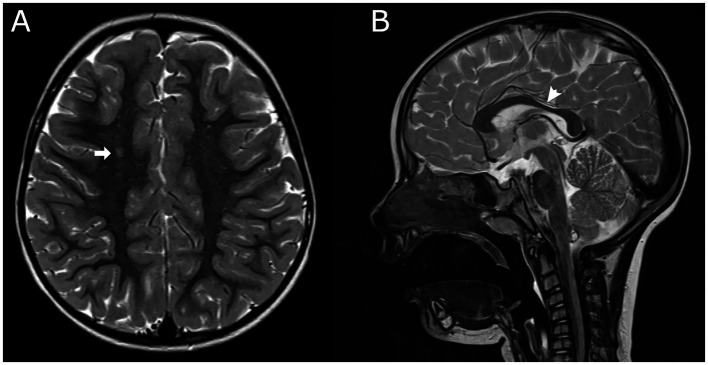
Brain MRI of Patient no. 1. (A) Axial T2-weighted image shows a small focus of increased signal in the right frontal centrum semiovale (arrow). Additional subtle white matter hyperintensities were present in the left peritrigonal region and right parietal subcortical white matter (not shown). (B) Sagittal T2-weighted image demonstrates mild thinning of the dorsal third of the corpus callosum (arrowhead). No additional pathological intracranial or spinal abnormalities were identified. The neuroradiological interpretation was consistent with mild chronic changes of presumed ischemic etiology. For data on perinatal history, see Table 4. Genetic testing revealed a *de novo* pathogenic variant in the ATL1 gene, consistent with a diagnosis of spastic paraplegia type 3A.
